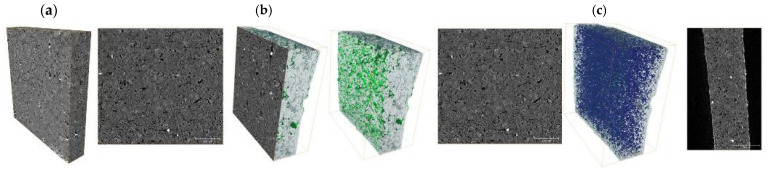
Results of X-ray tomography for a Nd sensitive hybrid membrane with 1 wt.% MWCNT-NdIIPs: (a) cross-section, (b) 3D model of bright phase, and (c) 3D model of pores and voids.
